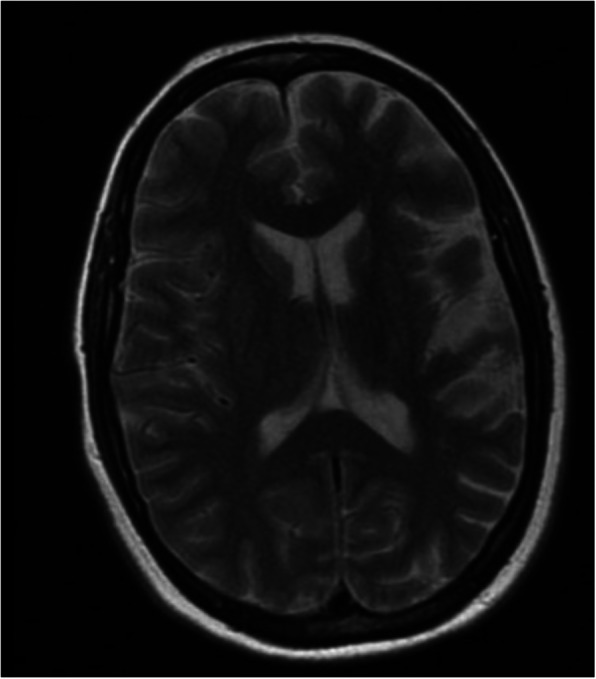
Mother (II-2) MRI brain showing T2 hyperintensity without enhancement in the deep and subcortical white matter of the left frontal lobe suggesting remote infarct in the left middle cerebral territory
